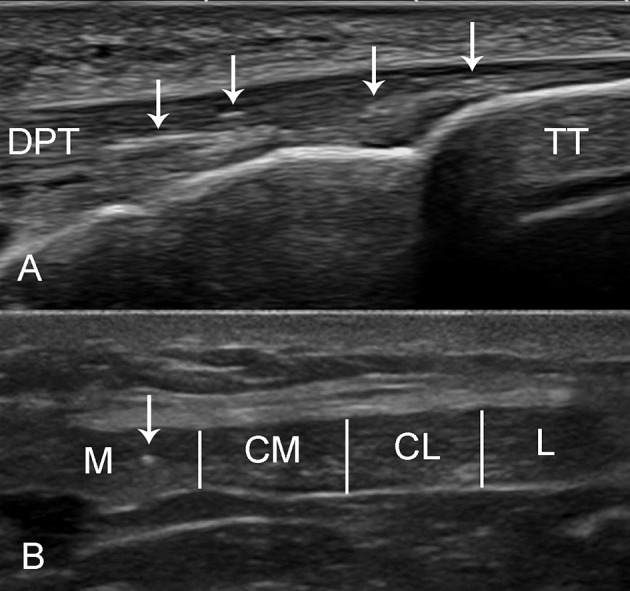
Ultrasound imaging of the distal patellar tendon (DPT). (A) The long axis of the DPT of a 34-year-old male was evaluated with Toshiba linear ultrasound probe (5–14 MHz). The bright foci (arrow) can be seen at the tendon insertion into the tibial tuberosity (TT). (B) The short axis of the patellar tendon (Mindray) was divided into four zones depending on the localization of the bright spots: medial (M), centro-medial (CM), centro-lateral (CL), and lateral (L). A small bright focus can be seen in the medial region. The patellar tendon appears hypoechoic because of anisotropy.
